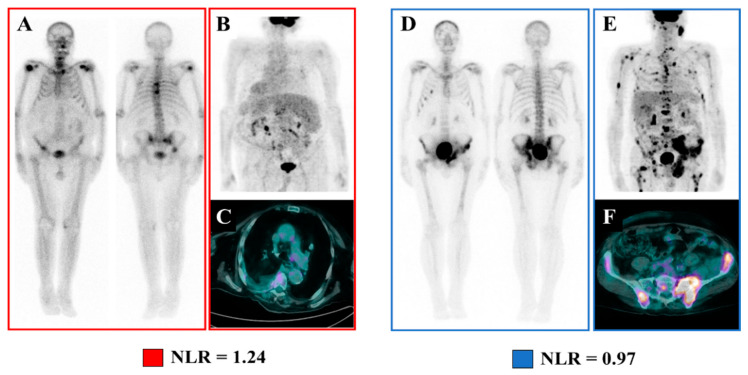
An emblematic example of a mismatch between the metabolic burden of metastatic disease and the systemic inflammation state. At baseline, these two patients showed similar degrees of metastatic burden at bone scan (Panels (A–D)) and similar NLR values, while FDG–PET showed a relevant mismatch in the extent of the metabolically active metastatic burden (Panels (B–E) show the Maximum intensity projection PET images, while Panels (C–F) show the axial section of the hottest bone lesion; MTV = 79.1 cm^3^ and 985.4 cm^3^, respectively).
